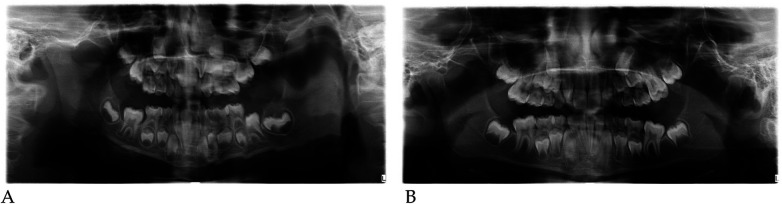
Pre- (A) and post-treatment (B) panoramic radiographs of case 4.
